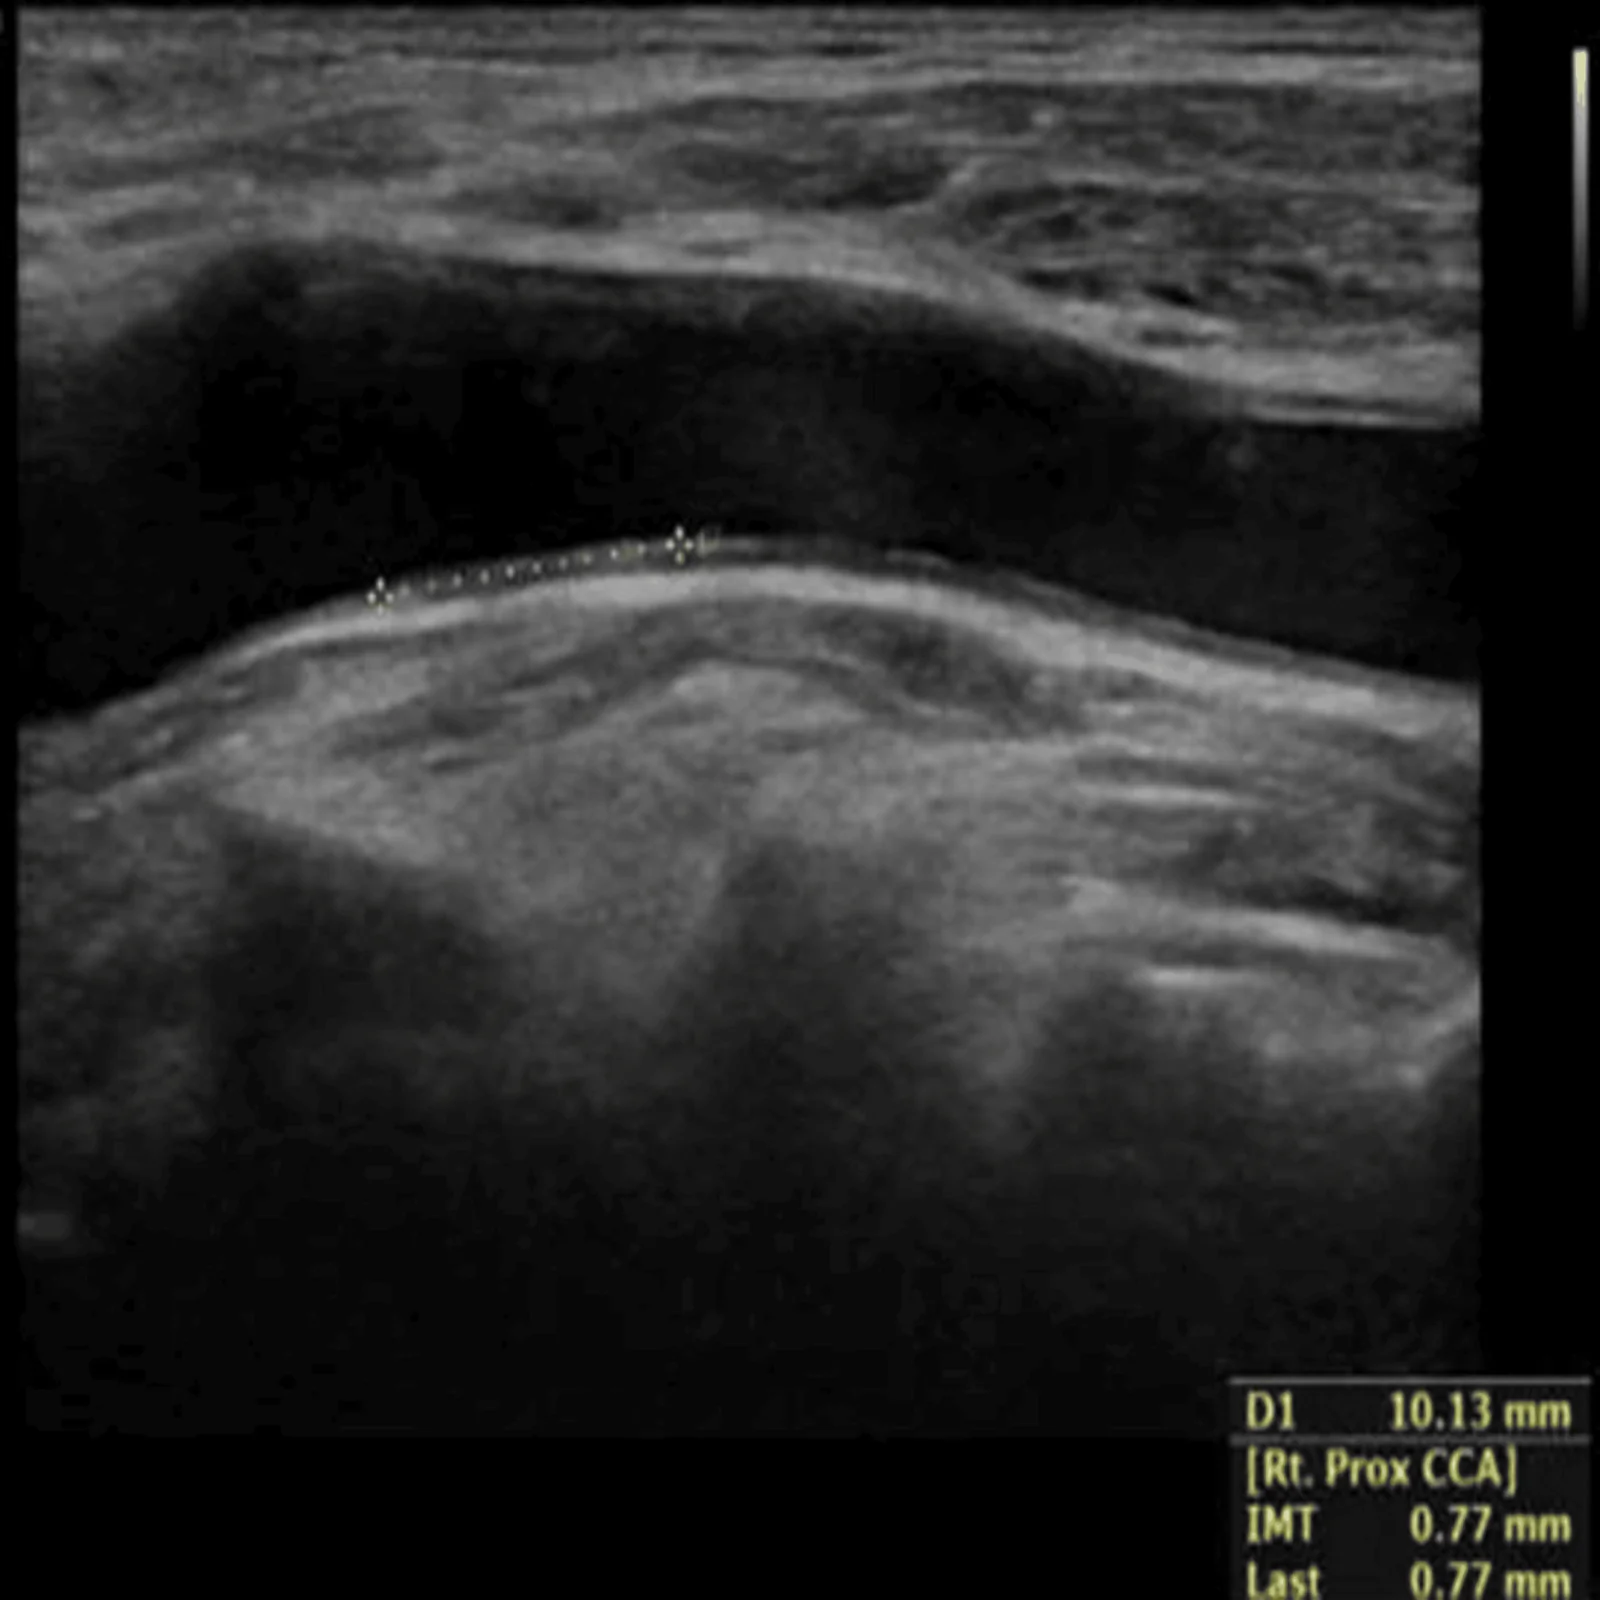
B-mode ultrasound of the right proximal common carotid artery demonstrating far-wall carotid intima media thickness measurement of 0.77 mm.
